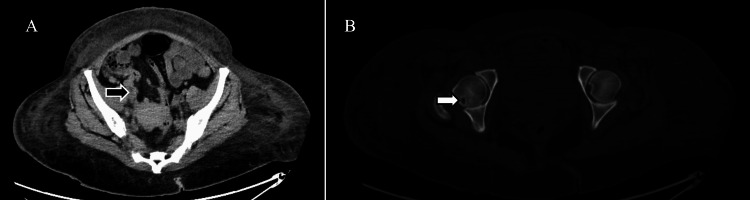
A CT scan shows evidence of metastatic mantle cell lymphoma. A: Representative image of lymphadenopathy (black arrow) measuring 1.6 cm; B: Lytic bone lesion (white arrow) measuring 0.8 cm.
